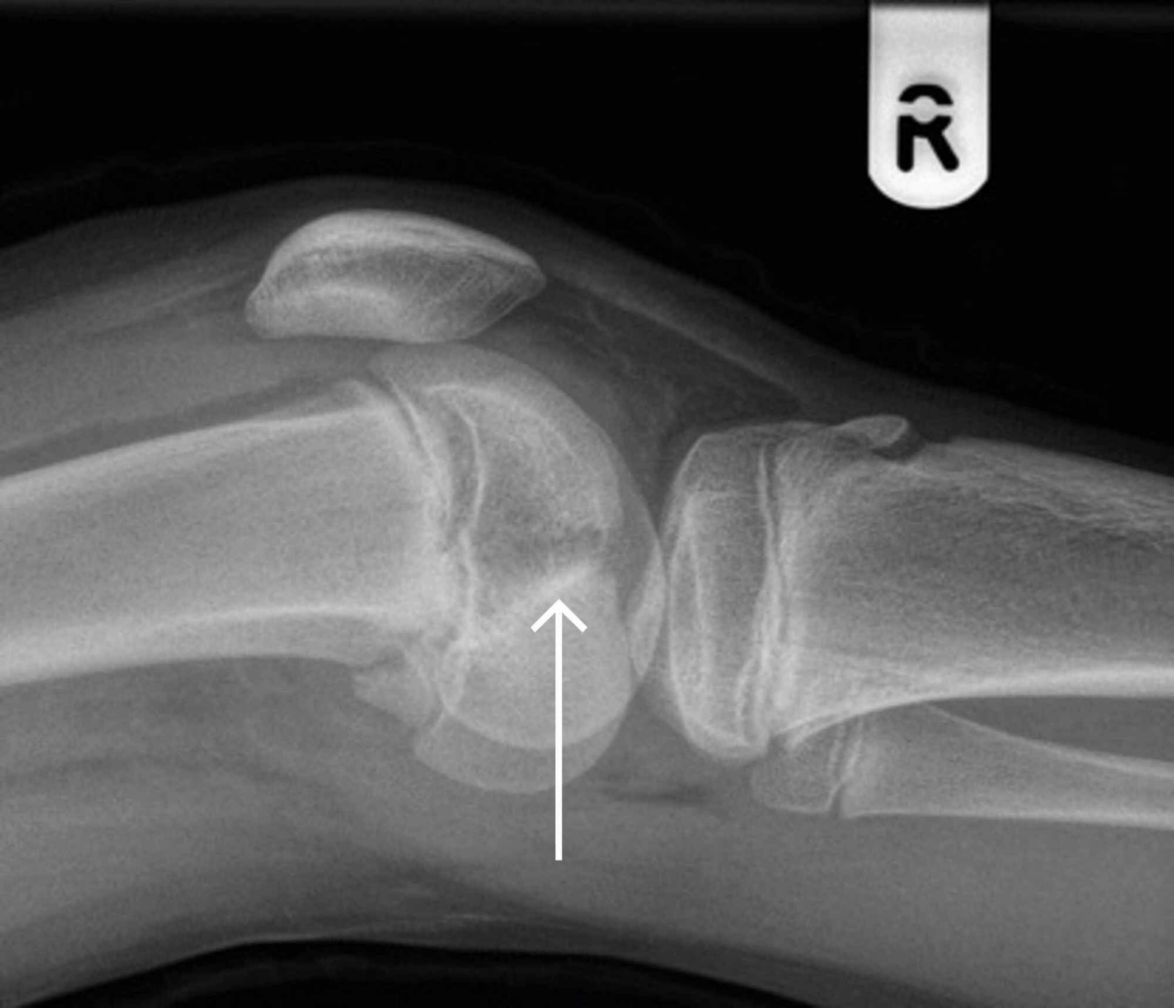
Lateral x-ray of right knee post-injury
